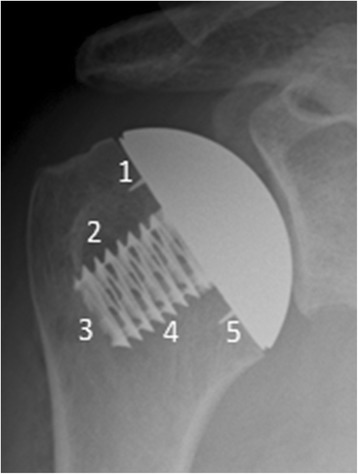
Anteroposterior radiographs of the stemless humeral head implant showing five radiographic zones including the superior and inferior interface (zone 1 and 5) and the interfaces around the hollow screw (zones 2, 3, and 4), which were used for the assessment of radiolucency and osteolysis
